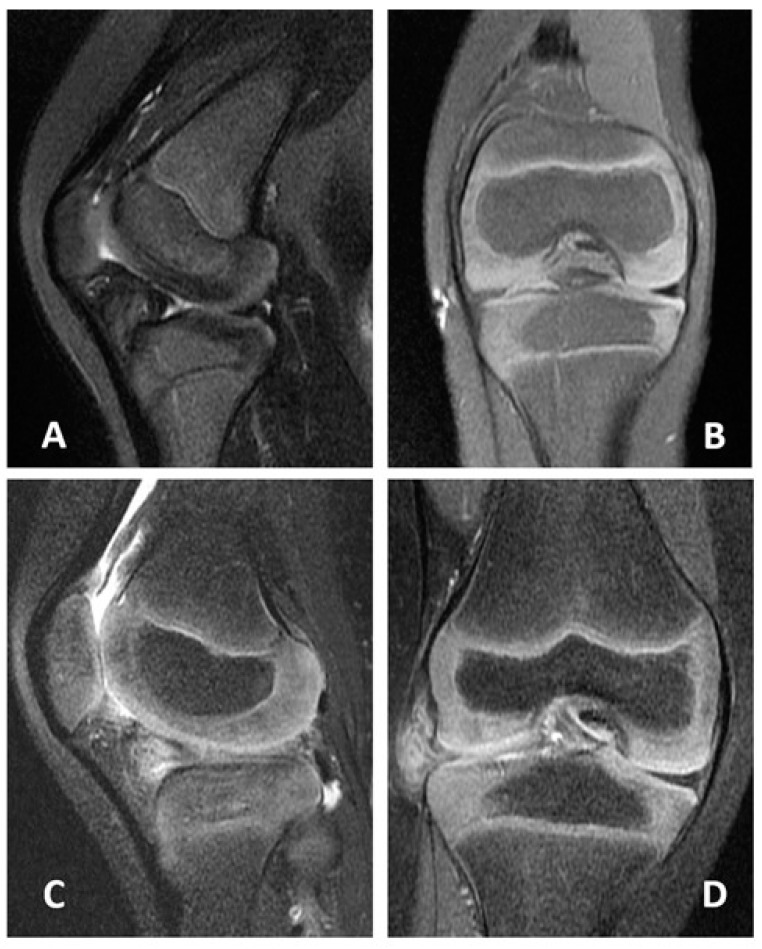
MRI of the affected knees for the two cases at the 6-month follow-up. (A) coronal and (B) sagittal planes for the case of the 28-month girl. (C) coronal and (D) sagittal planes for the case of the 5-year-old boy. A triangular lateral meniscus without a retear was found in both cases. MRI—magnetic resonance imaging.
